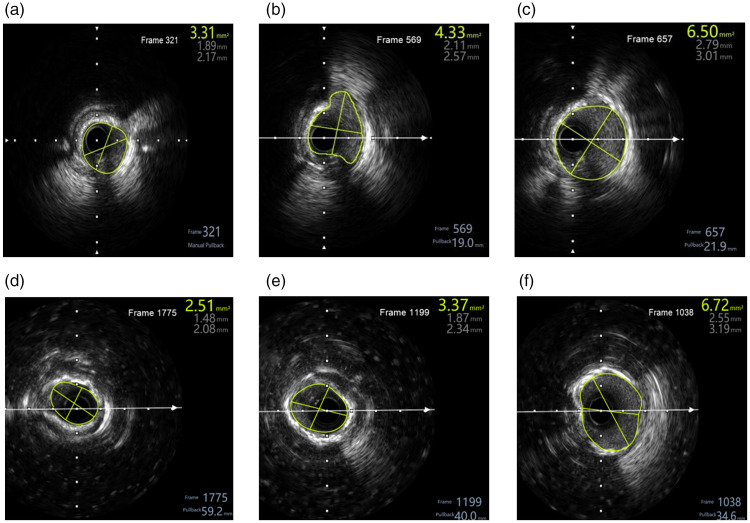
Intravascular ultrasound-guided intravascular lithotripsy and rotational atherectomy for coronary calcified lesions. (a) A ring of severe calcification was observed before intravascular lithotripsy treatment. The area was 3.31 mm^2^. (b) After intravascular lithotripsy, this area was 4.33 mm^2^. (c) The final treatment area was 6.50 mm^2^, and the stent was well expanded and adhered to the wall. (d) A ring of severe calcification was observed before rotational atherectomy treatment. The area was 2.51 mm^2^. (e) After rotational atherectomy, this area was 3.37 mm^2^ and (f) the final treatment area was 6.72 mm^2^, and the stent was well expanded and adhered to the wall.
